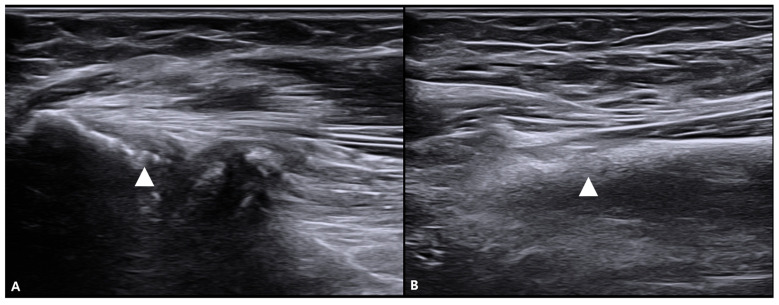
Proximal sonographic findings in the tendon-dominant phenotype. Ultrasound images demonstrate focal calcific/enthesopathic changes at the proximal flexor compartment within the common flexor origin region. (A) Calcification (white arrowhead) is visualized at the proximal origin region of the flexor digitorum superficialis (FDS) in the forearm. (B) Corresponding calcification (white arrowhead) is seen at the origin region of the flexor digitorum profundus (FDP). These proximal findings exemplify the expanded anatomical scope of assessment in the proposed origin-to-insertion framework; when accompanied by power Doppler activity or other inflammatory features, an inflammatory enthesitis phenotype (e.g., psoriatic arthritis) should be considered in the differential diagnosis.atic arthritis) when accompanied by power Doppler activity or other inflammatory features.
